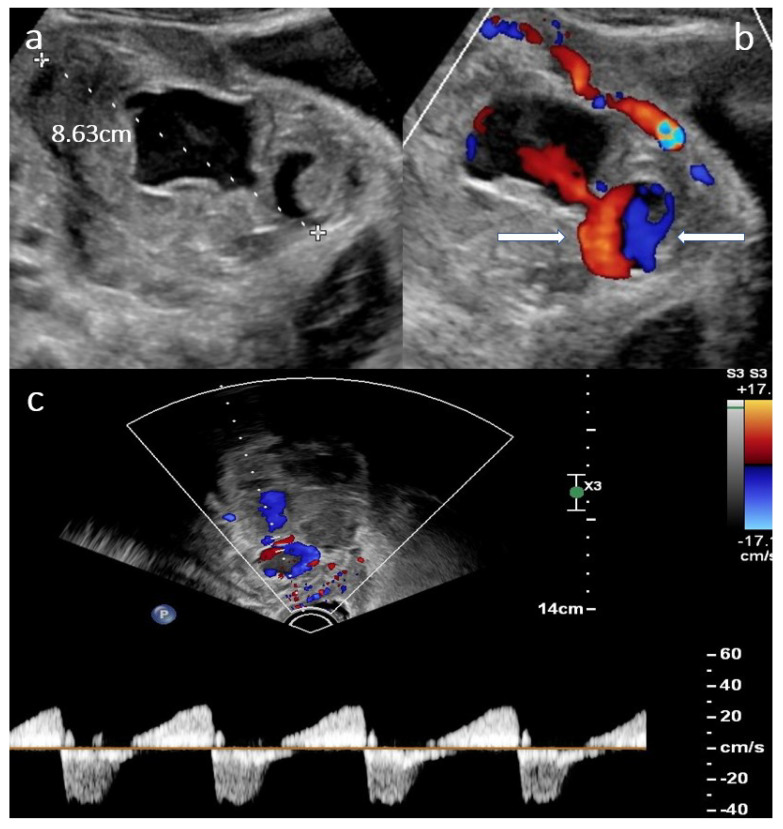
(a) Ultrasound findings in B-mode showing an anechoic lesion within an echogenic mass representing the UAP with surrounding hematoma. (b) Color Doppler showing Yin–Yang sign (between arrows). (c) Pulsed wave Doppler showing a to-and-fro pattern in the lower part of the image.
